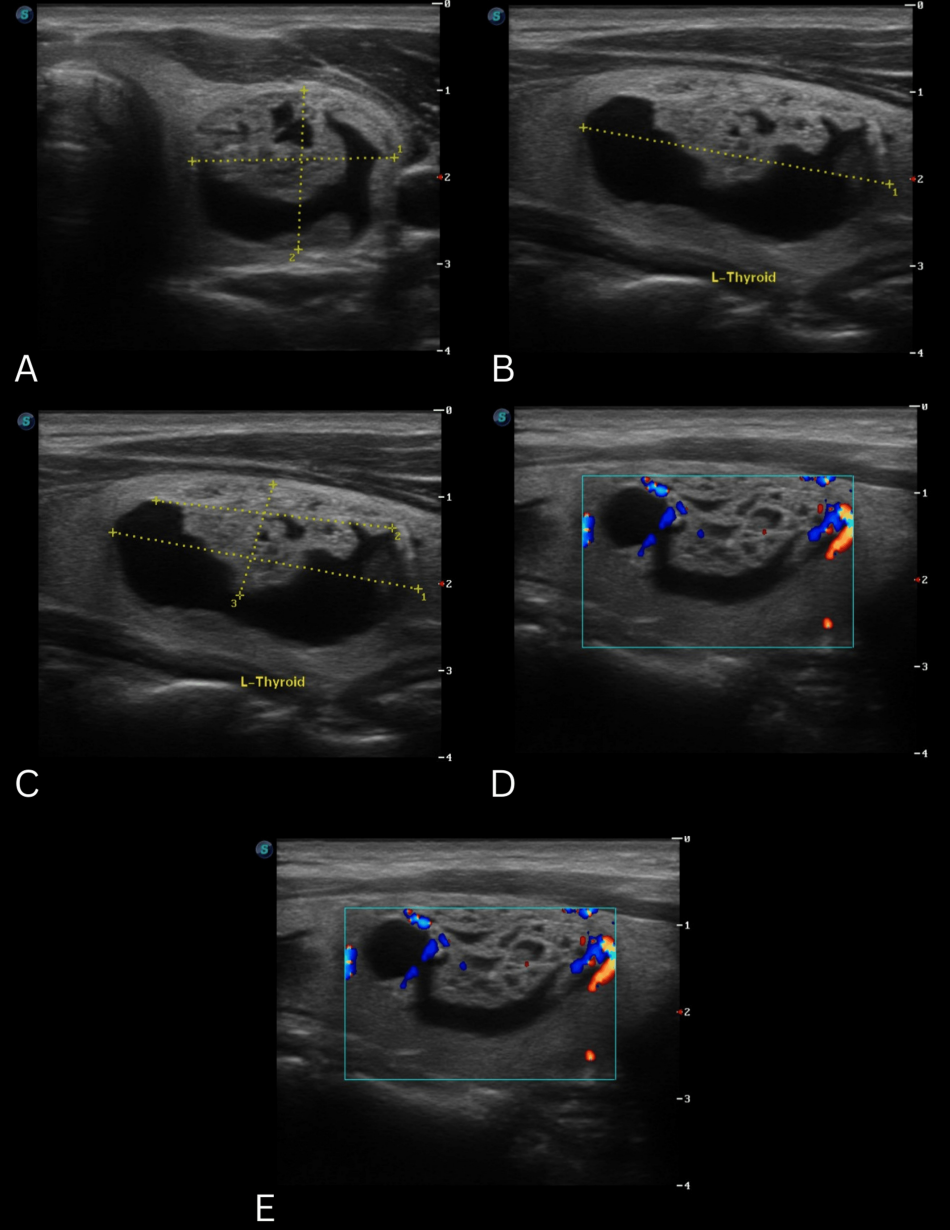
Clinical images series of TUSG 1 A. Nodular lesion in the left thyroid lobe, mixed echogenicity with a cystic predominance (1), the margins of the lesion are regular, and the margins of the solid component are non-spiculated (0); B. Wider-than-tall (0); C. Solid component isoechoic (1), with no hyperechogenic foci (0); D. Peripheral vasculature with color Doppler, short axis; E. Peripheral vasculature with color Doppler, long axis; TIRADS-2 (2 points) 27.43 x 13.31 x 35.84 mm TSUG: thyroid ultrasonography; TIRADS: Thyroid Imaging Reporting and Data System
